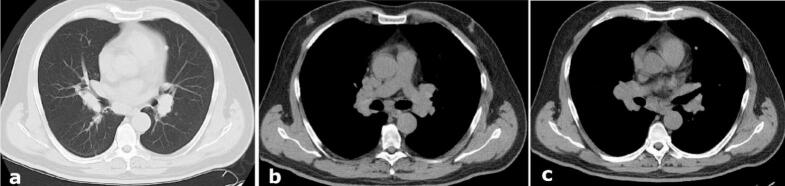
(a–c) Chest Computed tomography (CT) images showing bilateral hilar lymphadenopathy and interstitial nodular opacities suggestive of pulmonary sarcoidosis.
